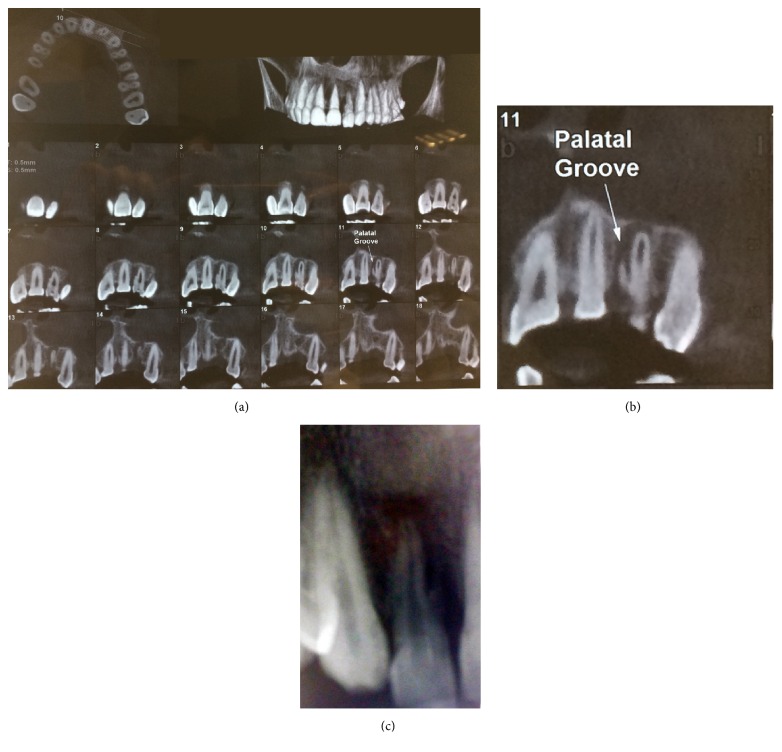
(a) Preoperative cone beam computed tomography (CBCT); (b) CBCT revealed a radicular groove on tooth #10; (c) preoperative periapical radiograph, there is a large radiolucency in relation to left lateral incisor.
